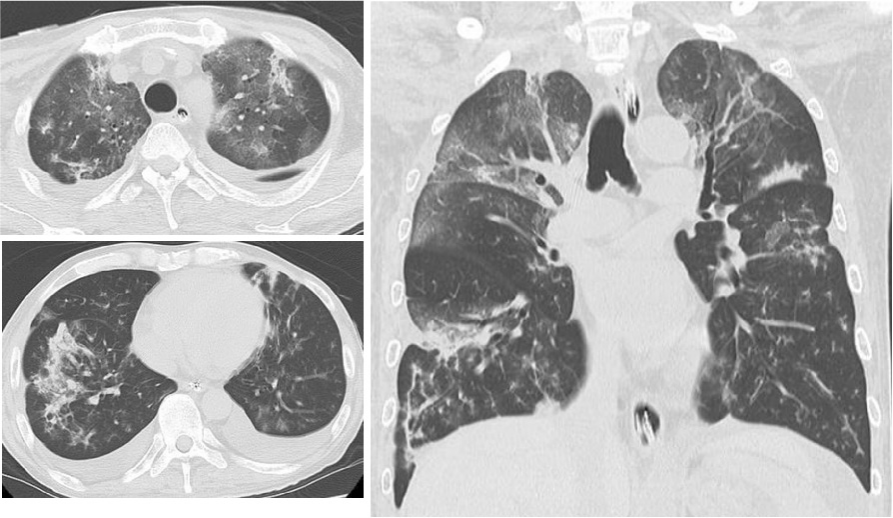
Computed tomography scans of the patient taken on the 12th ICU day. These images show the amelioration of the consolidations of the bilateral lung fields.
